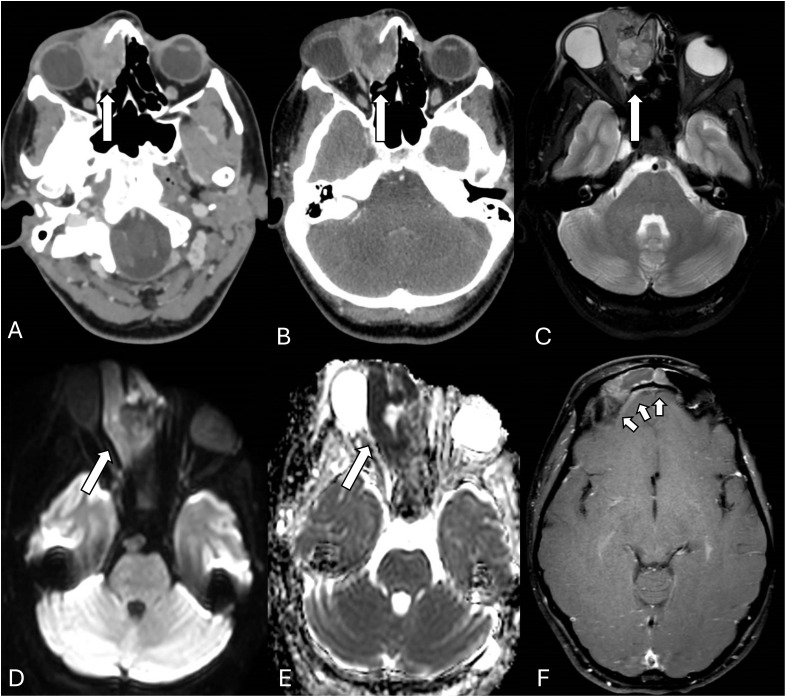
Contrast-enhanced computed tomography of the brain at initial imaging work-up (A) and 14 days later (B). Right orbitoethmoidal mass (arrows) initially showing heterogeneous but mostly enhancing solid tissue with contiguous invasion of the medial aspect of the right orbit. Note the extensive osteolysis on the medial orbital wall, invasion of extraconal fat, and close contact with the medial rectus muscle with subtle sparing of the fat plane. (B) shows significant mass enlargement and extensive low-enhancing central areas that were not present in (A), with increasing mass effect on the right ocular globe, which shows loss of sphericity and is displaced laterally. (C-F) Axial MR images two days after (B) [(C), T2-weighted; (D), diffusion-weighted image –b=1500-; (E), ADC map; (F), Post-contrast T1-weighted]. The mass shows heterogeneous but predominantly low T2 signal intensity, as well as high DWI signal and low ADC values consistent with significant diffusion restriction. (F) shows thickening and increased enhancement of the dura mater below the right frontal sinus, consistent with dural invasion [short arrows in (F)]. ADC, apparent diffusion coefficient; DWI, diffusion-weighted imaging; MR, magnetic resonance.
